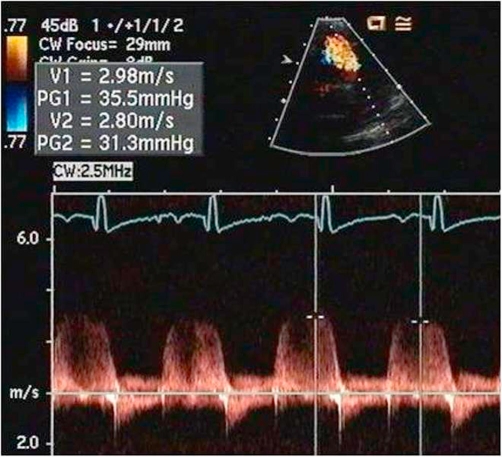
Spectral Doppler interrogation of the pulmonary regurgitant jet showing the pulmonary artery diastolic pressure to be elevated at 35 mmHg above the right ventricular end diastolic pressure.
